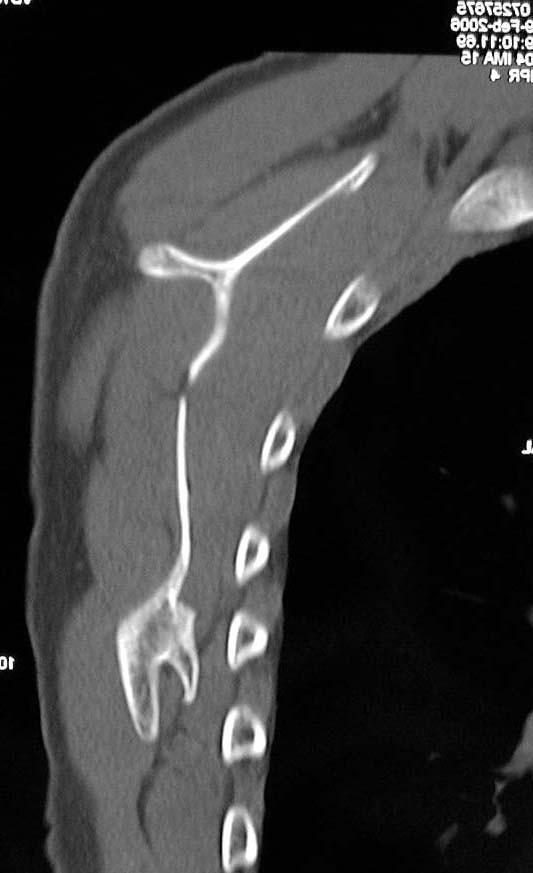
CT image shows an osteochondroma on the anterior and inferior surface of the right scapula.
